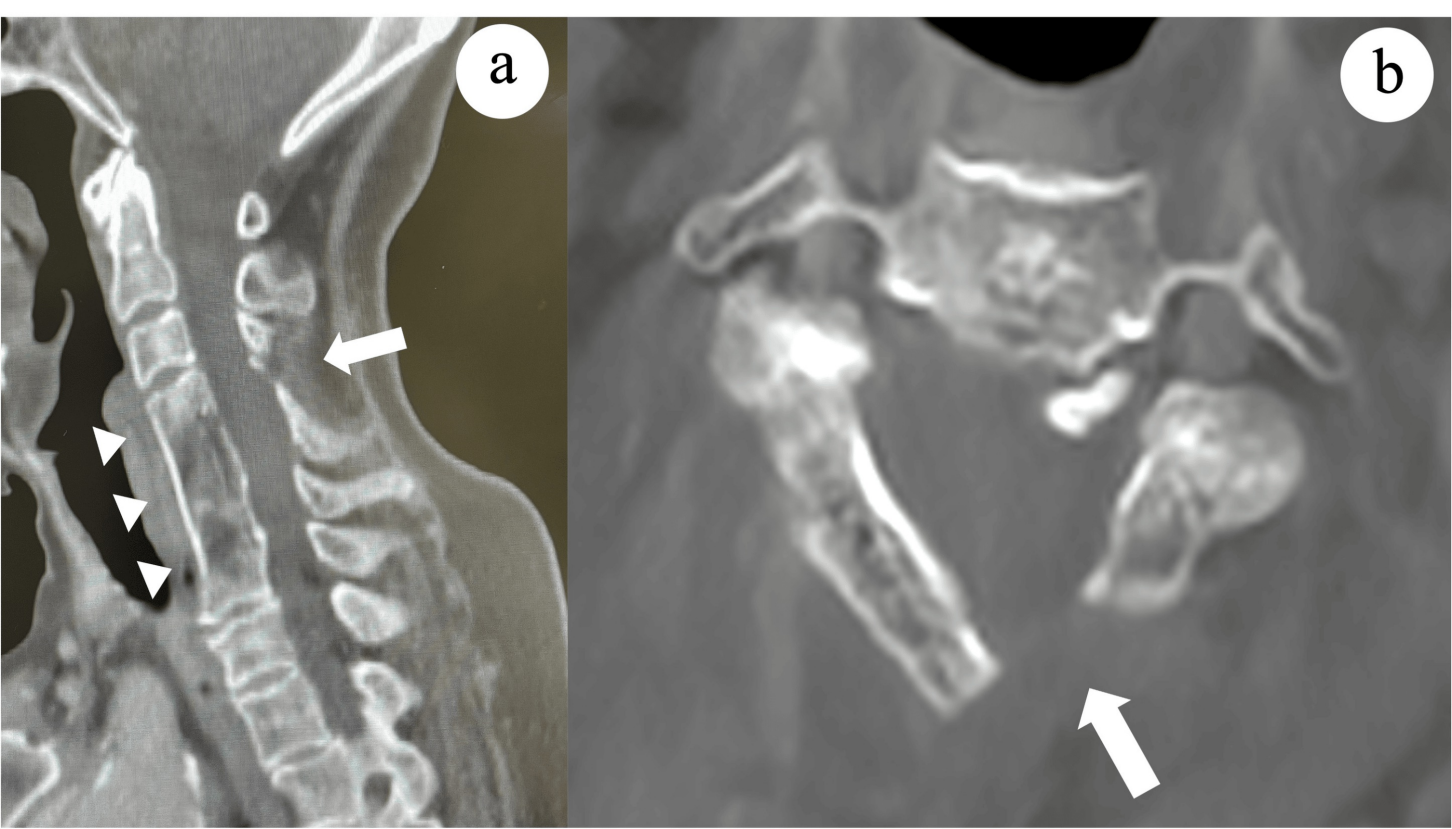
Radiological findings before fracture. (a) Sagittal and (b) axial computed tomography scans obtained 20 years earlier showing anterior corpectomy and fusion surgery at C4-C7 with an iliac autograft (arrowheads) and open-door cervical laminoplasty at C4 (arrows) performed for cervical spondylosis and radiculopathy.
